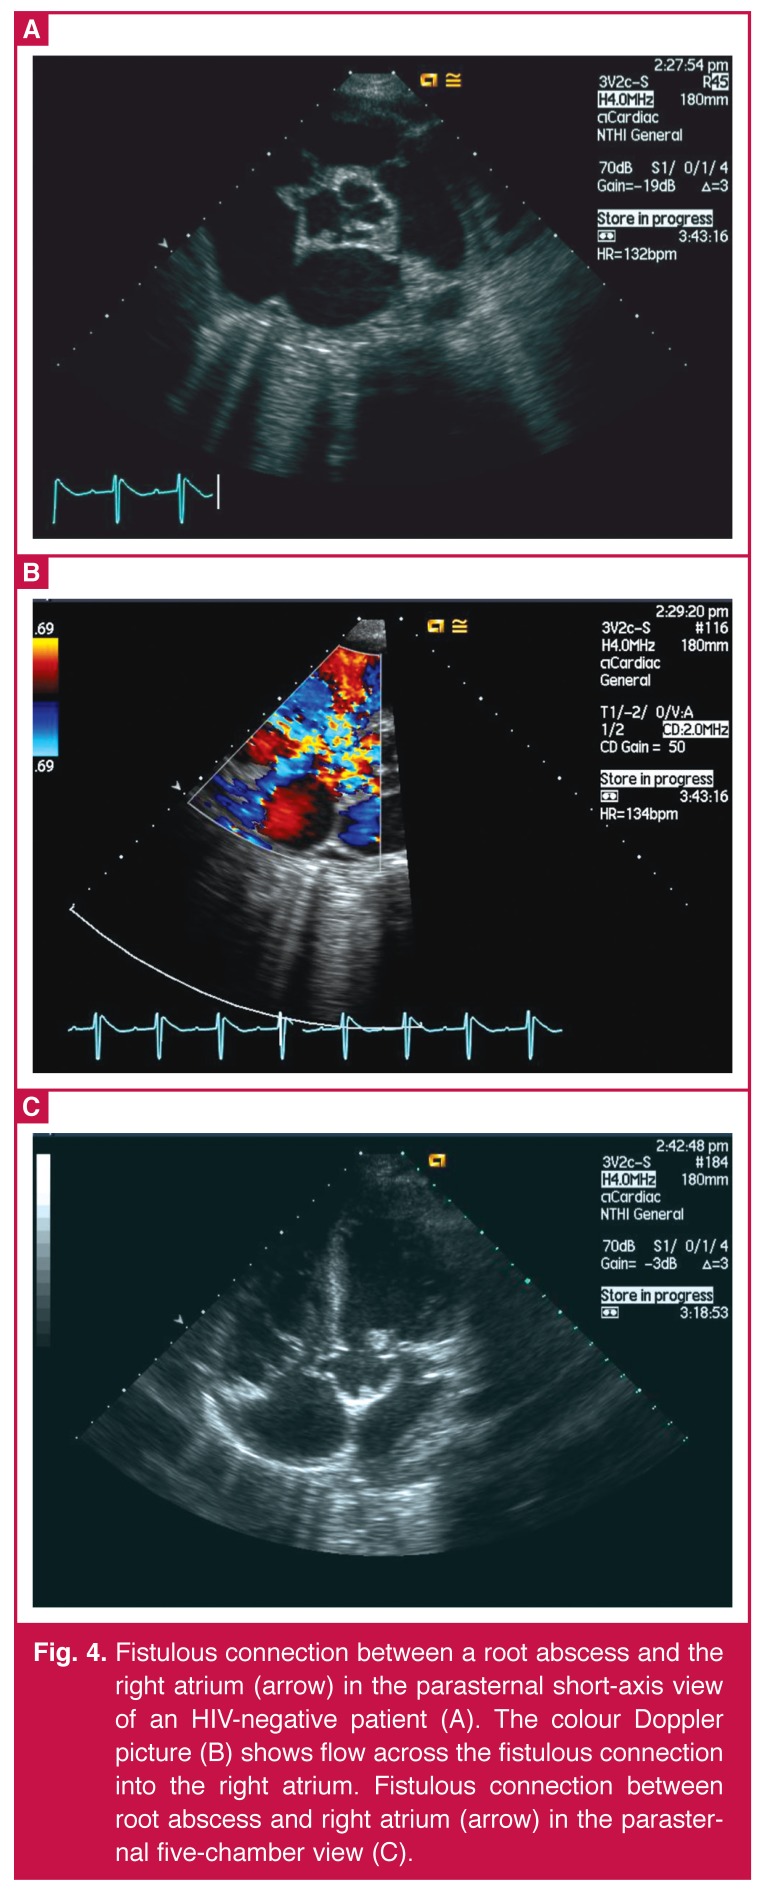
Fistulous connection between a root abscess and the right atrium (arrow) in the parasternal short-axis view of an HIV-negative patient (A). The colour Doppler picture (B) shows flow across the fistulous connection into the right atrium. Fistulous connection between root abscess and right atrium (arrow) in the parasternal five-chamber view (C).
